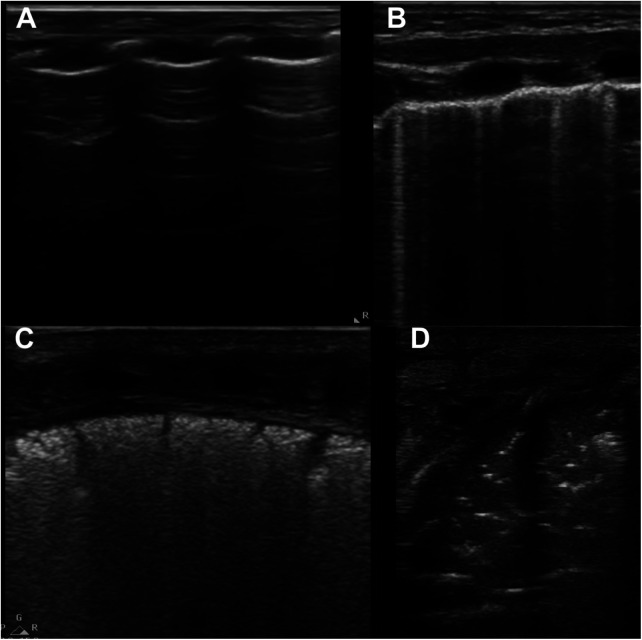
Lung ultrasound patterns used to calculate lung ultrasound scores. (A) score 0, A-pattern (defined by the presence of only A-lines); (B) score 1, B-pattern (defined as the presence of ≥3 B-lines); (C) score 2, severe B-pattern (defined as the presence of crowded and coalescent B lines with or without consolidations limited to subpleural space); (D) score 3, extended consolidation. Modified with permission from Corsini et al. (31).
